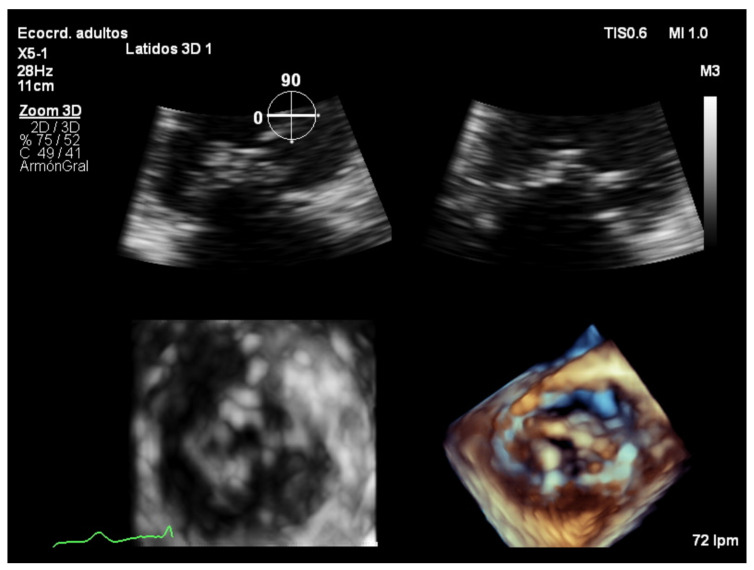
Zoom 3D focused on the mitral valve from the apical four chamber view.
